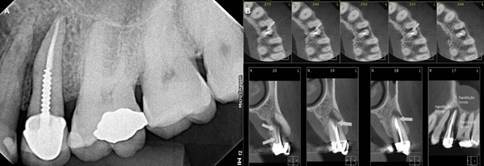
Example of one of the clinical cases. (A) Periapical radiograph of tooth 25. (B) CT scans provided for this case.
